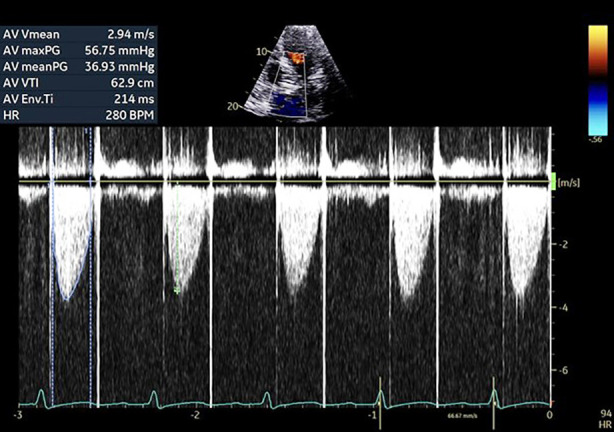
Echocardiography demonstrating increased aortic valve gradients and velocity.
